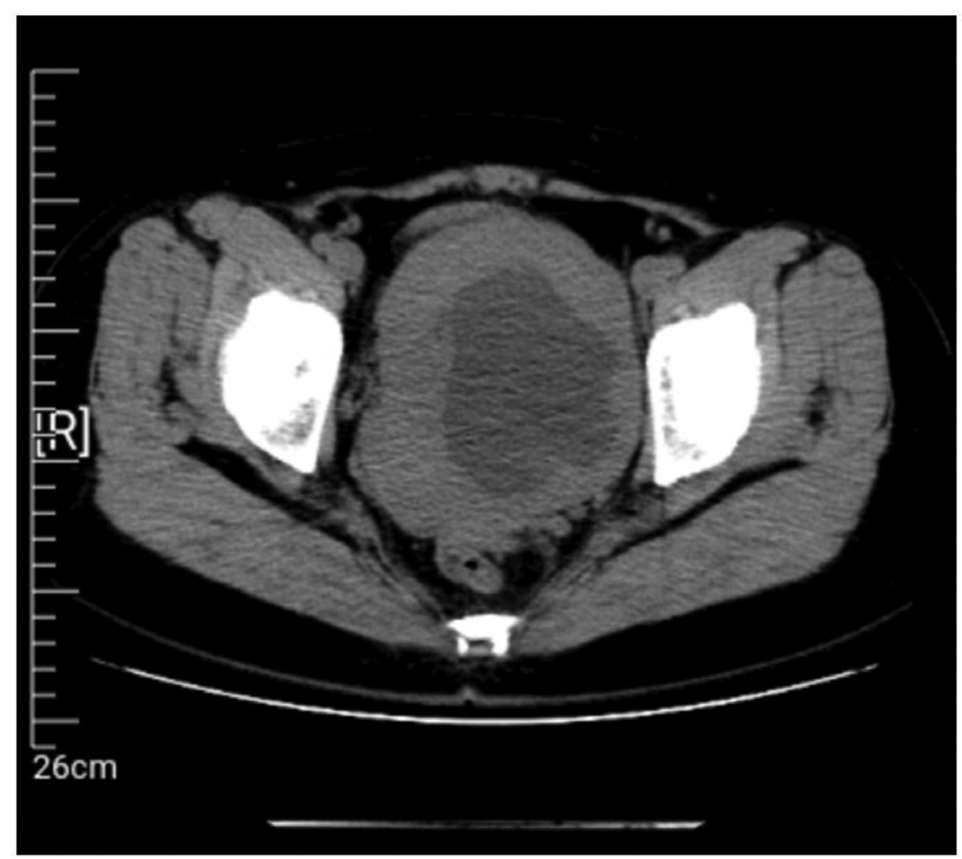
Pelvic computed tomography image showing an unclear defined 11 cm pelvic mass without further abnormalities.
